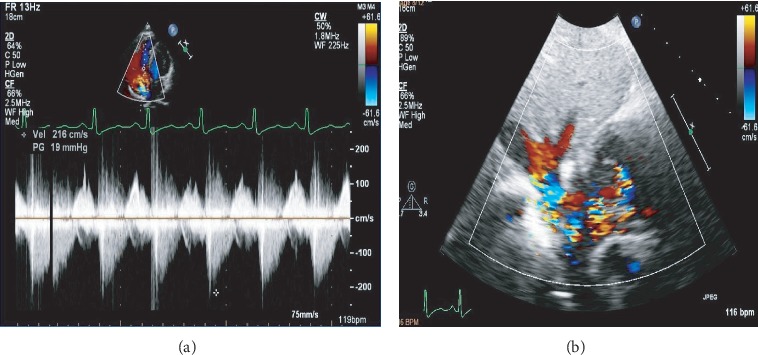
(a) Continuous wave Doppler through the tricuspid valve in 4-chamber view showing an early peaking triangular tricuspid regurgitation velocity. (b) A subcostal view showing hepatic vein systolic flow reversal due to severe tricuspid valve regurgitation on colour flow imaging.
